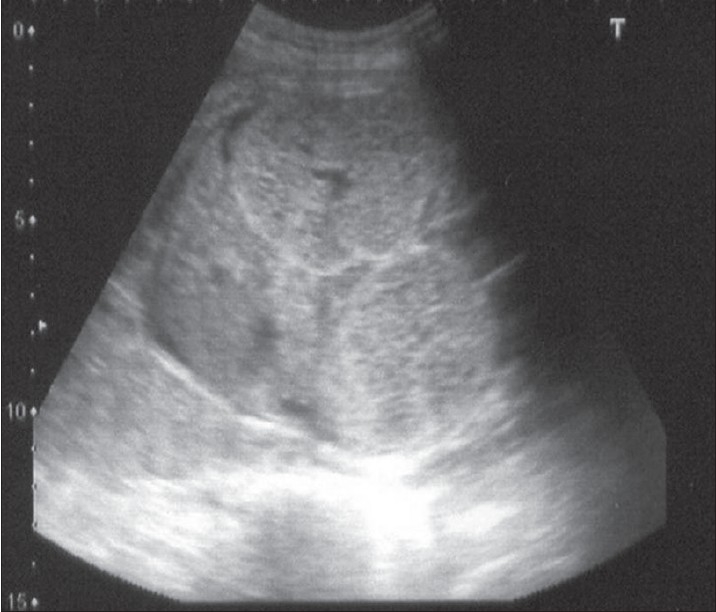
Antenatal ultrasound at 24 weeks showing bilateral, symmetrically enlarged, echogenic kidneys filling the fetal abdomen. Liver is normal
